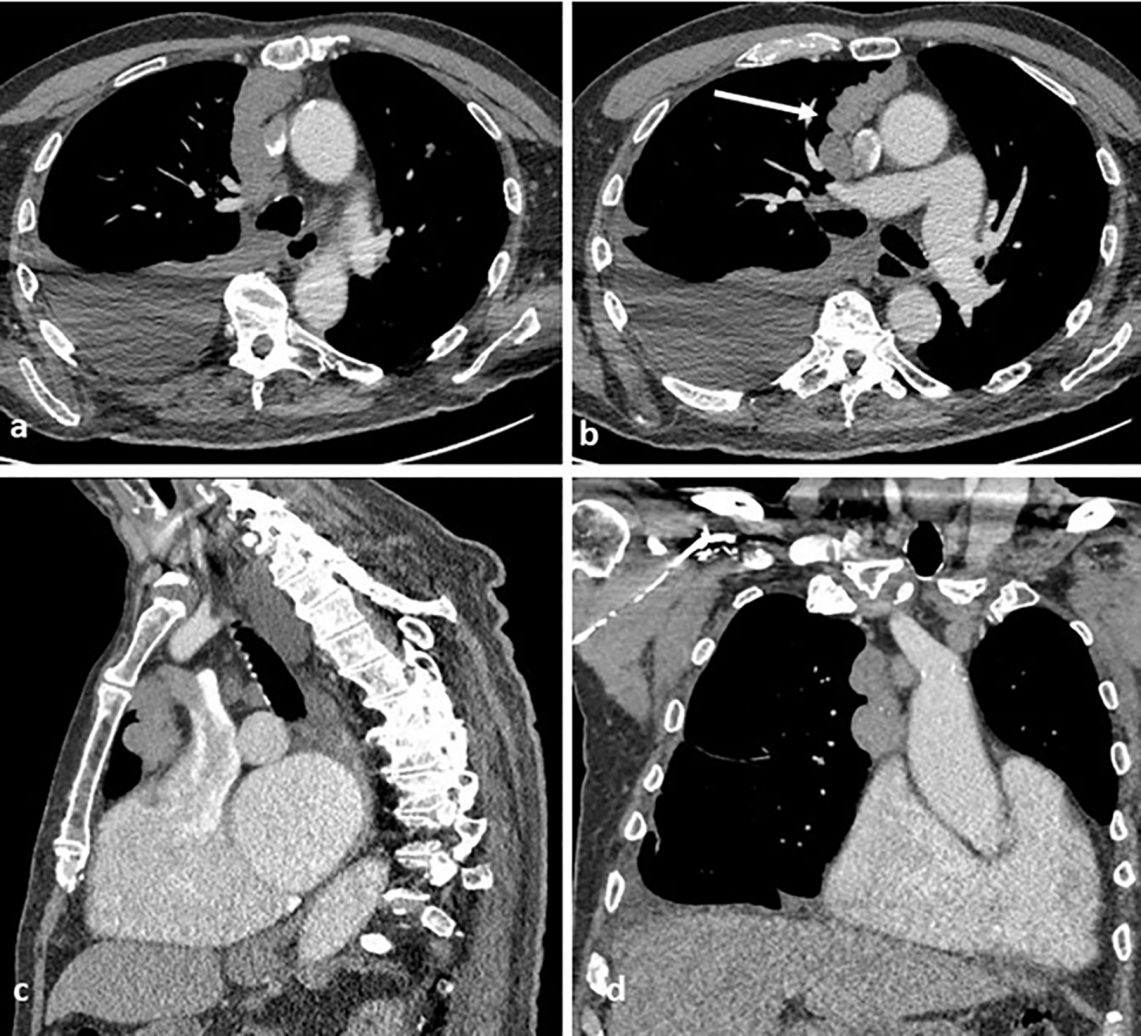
Images from preoperative computed tomography ([a] and [b]) show a right paramedian anterior mediastinal mass, with local involvement of the anterior aspect of the pericardium ([c] and [d]).
